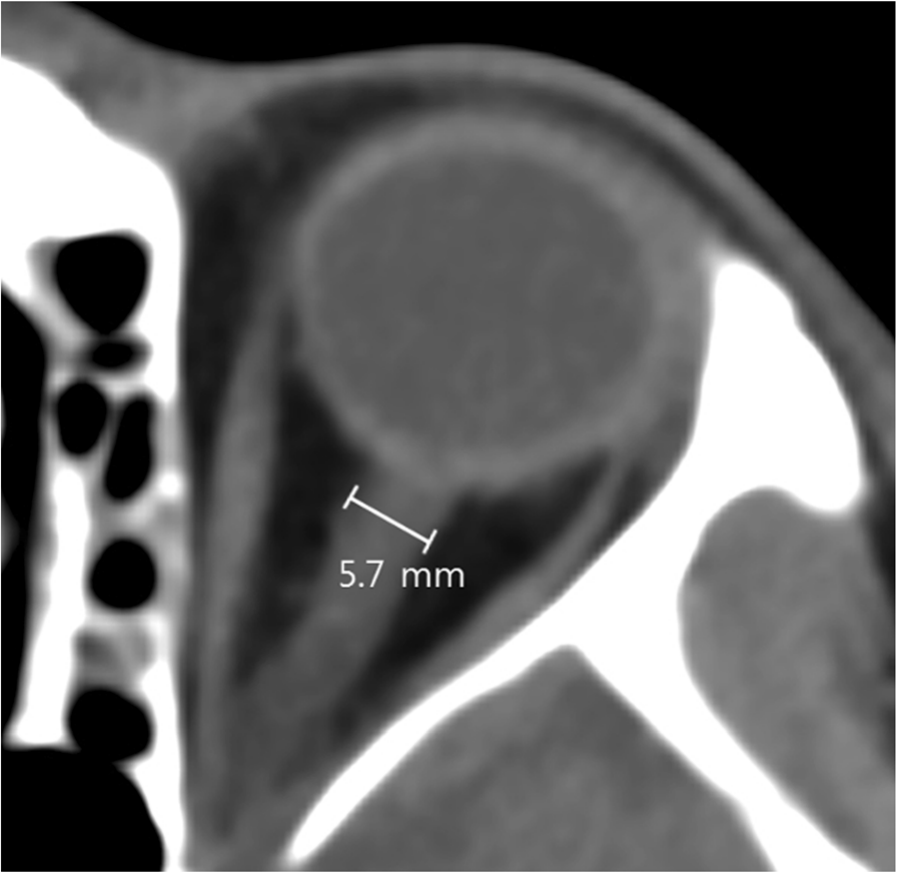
Measurement of optic nerve sheath diameter on initial brain computed tomography scan
